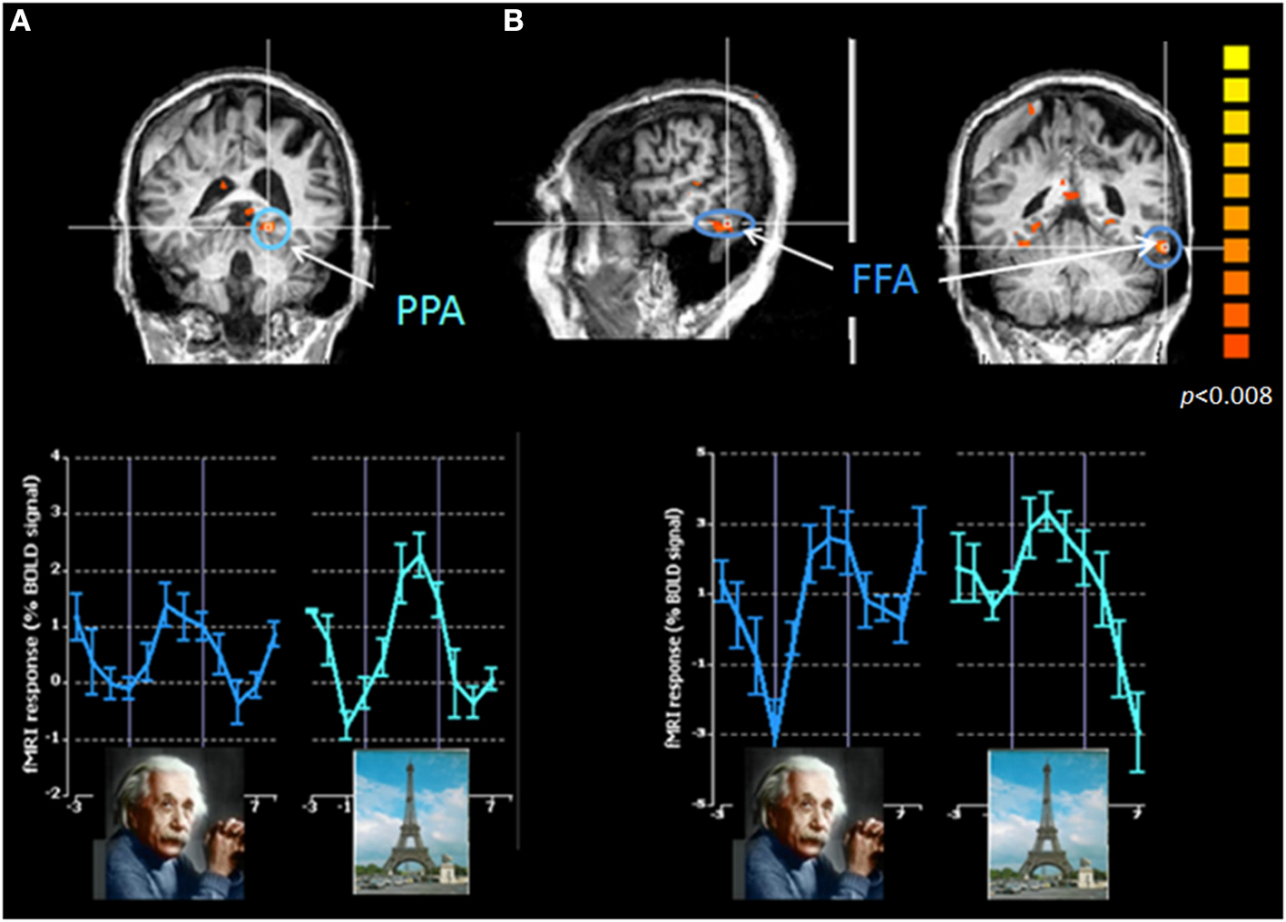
Cortical activation patterns during faces and places recognition in a patient with acquired visual cortical damage: Correspondence between behavioral and cortical dysfunctions. Cortical activation in the parahippocampal place area, PPA (A) and in the fusiform face area, FFA (B) during viewing pictures of faces and places. Upper panels represent the cortical activation maps centered on the two regions of interest (ROIs) (PPA marked in green, FFA marked in blue). Lower panels represent the activation elicited in each of these ROIs for the two stimuli type. Color scale denotes significance levels. The absence of cortical specification for processing faces corresponds to the patient's inability to process the identity of faces (while ability to process the identity of other visual stimuli is intact). MRI slices are shown in neurological convention.
